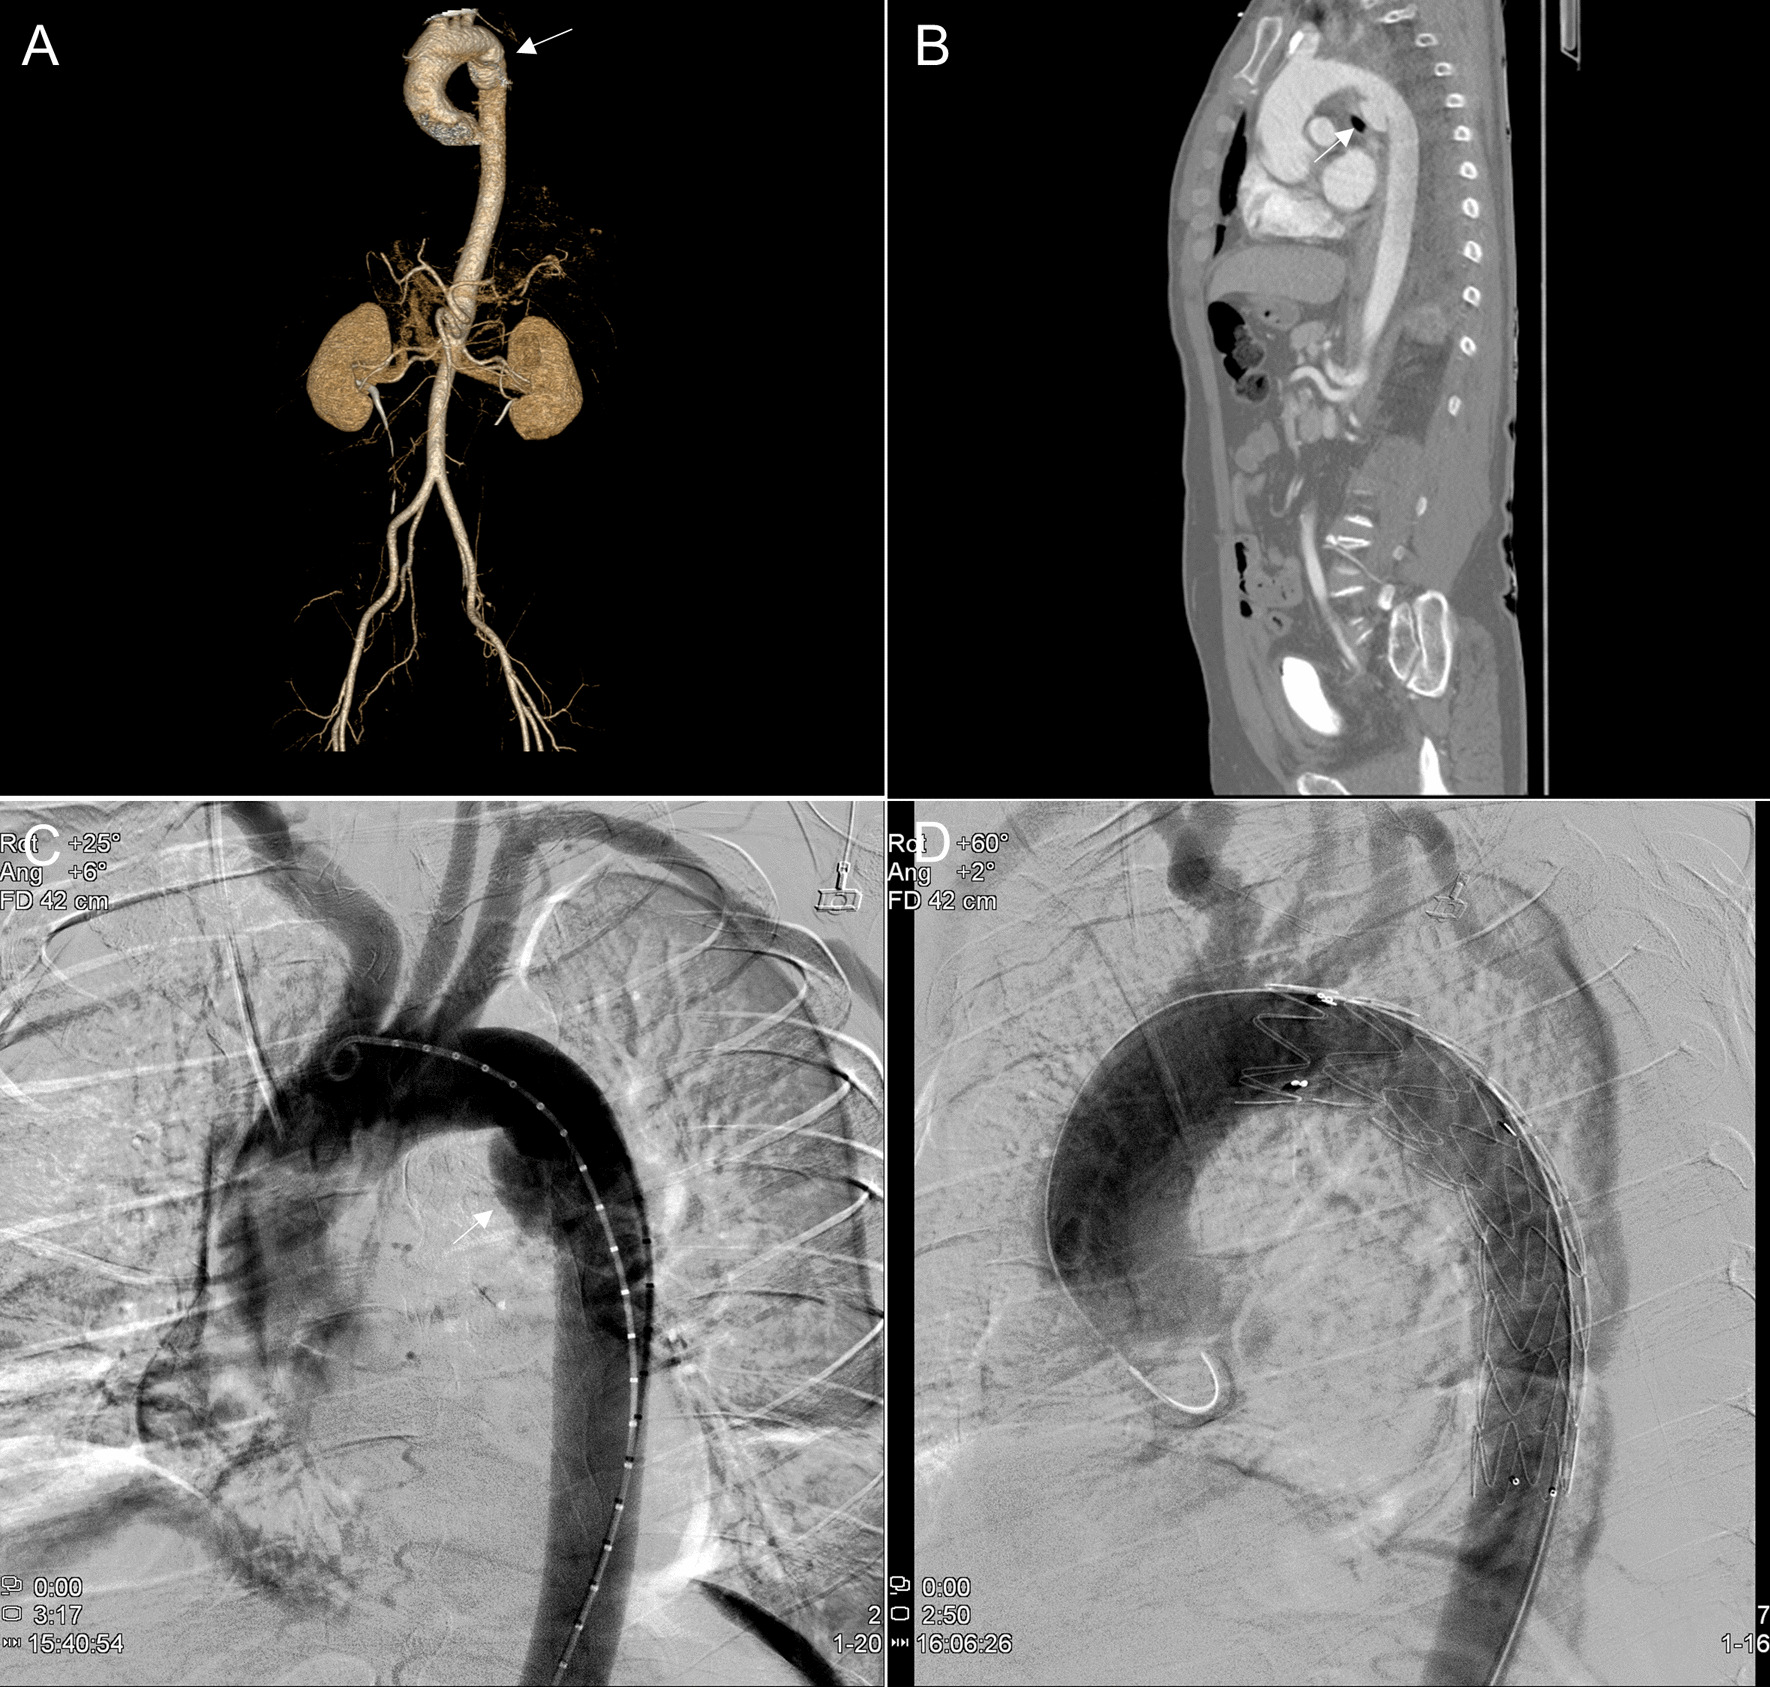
Computed tomography of aorta and aortogram of patient and aortogram performed after stent graft insertion. A 3D reconstruction image of patient’s aorta computed tomography. B Sagittal image of patient’s aorta computed tomography. C Aortogram of patient’s aorta. D Aortogram performed after stent graft insertion. White arrow indicates saccular dissection of descending thoracic aorta
